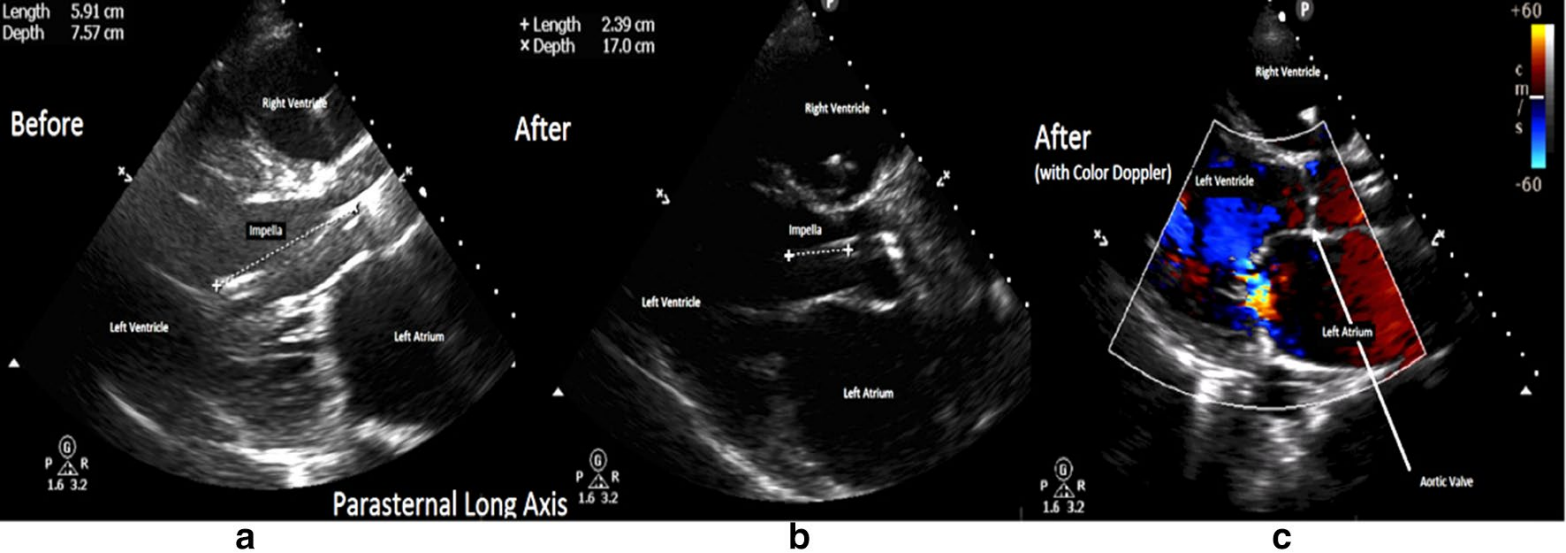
Impella positioning visualized on the parasternal long-axis view on the TTE exam. a Images acquired at the time of improper positioning. The Impella^®^ (hyperechoic structure) is located 5.9 cm beyond the aortic valve in the left ventricle. b Acquired after repositioning. Notice that the length of the Impella^®^ in the left ventricle is now 2.4 cm beyond the aortic valve into the LV. c Acquired after repositioning with color Doppler. Flow at the outflow area can be visualized. The outflow area is now located above the aortic valve
